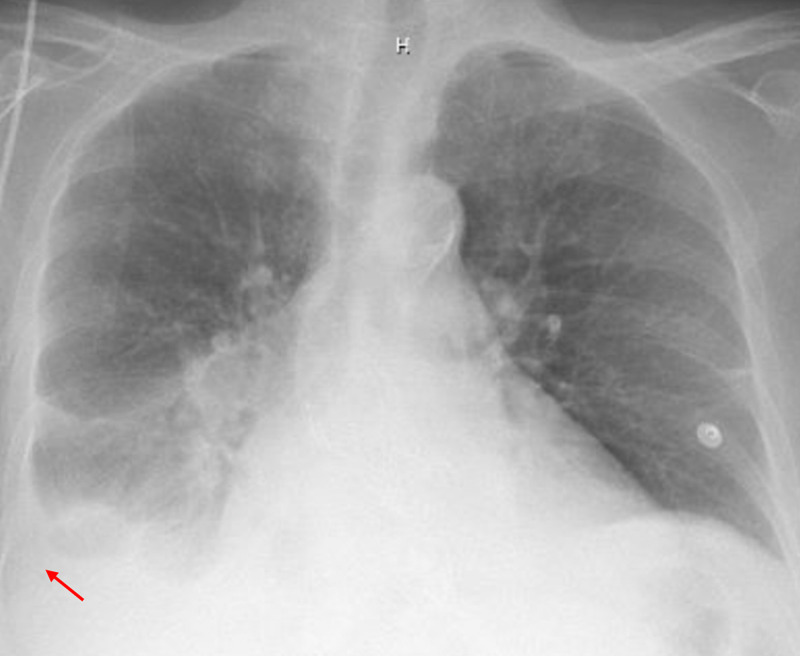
Chest X-ray depicting right-sided pleural effusion (red arrow)
